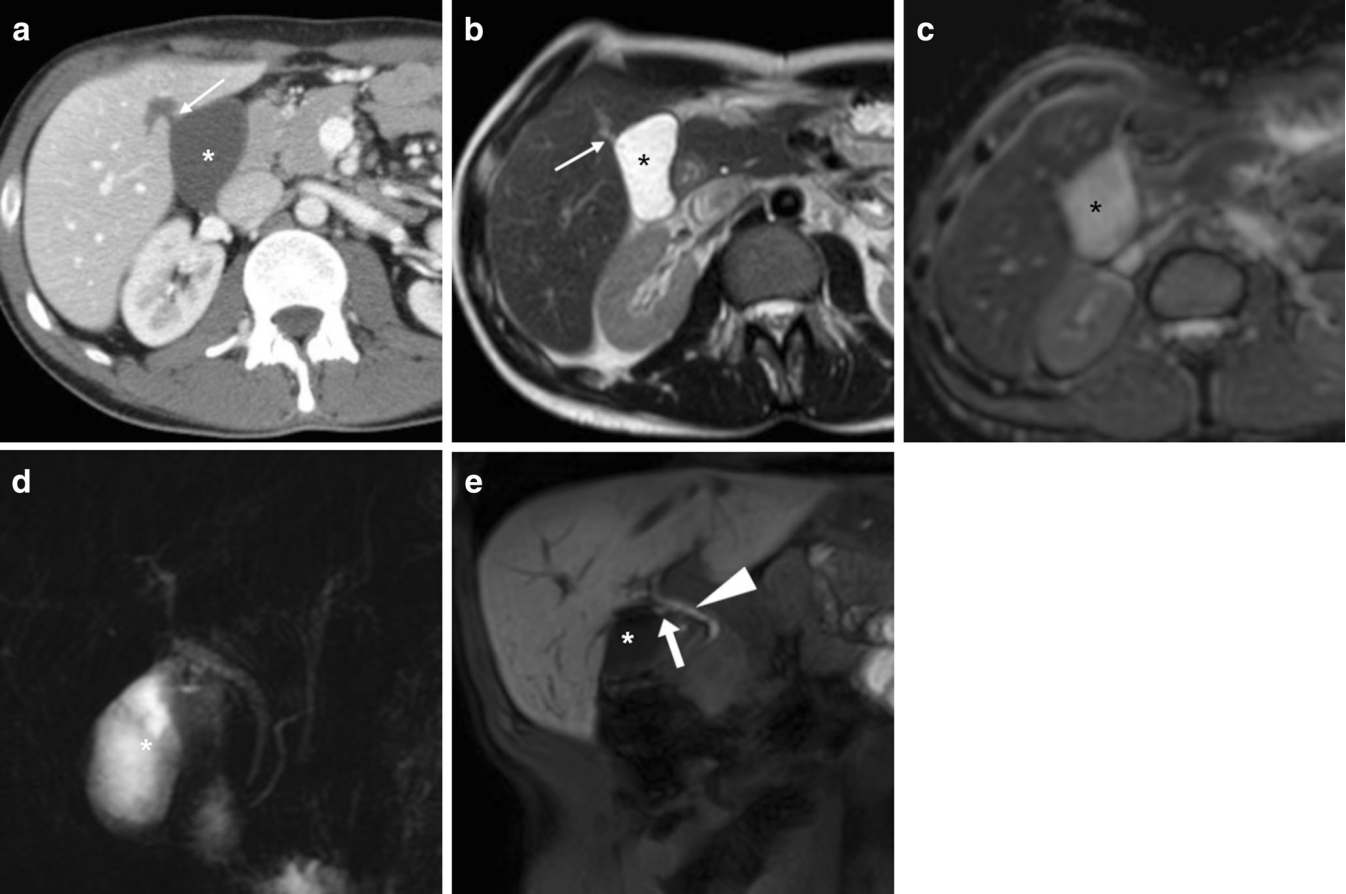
Typical biloma (*) observed 2 weeks after laparoscopic cholecystectomy as water-attenuation collection abutting the gallbladder fossa at CT (a). Some days later, MRI showed unchanged shape and size of the biloma, with homogeneous fluid and unrestricted diffusion on T2-weighted (b), apparent diffusion coefficient map (c) and MRCP (d). A small fluid track (*thin arrows*) was seen connecting the biloma to the hepatic parenchyma. Coronal (e) gadoxetic acid-enhanced MRCP image showed well-opacified bile in the common bile duct (*arrowhead*) and short cystic duct remnant (*arrow*), and no filling of the biloma, which was attributed to a sealed leak from small peripheral bile radicle
